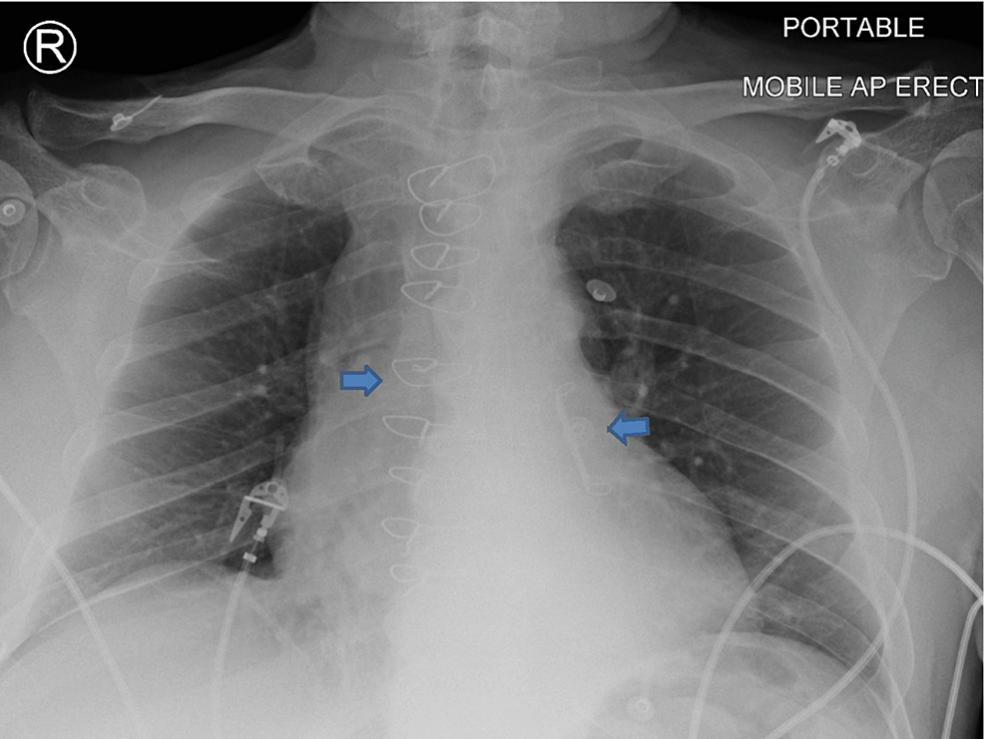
Antero-posterior chest radiography showing sternotomy sutures, and left atrial appendage closure device (pointed arrows).
